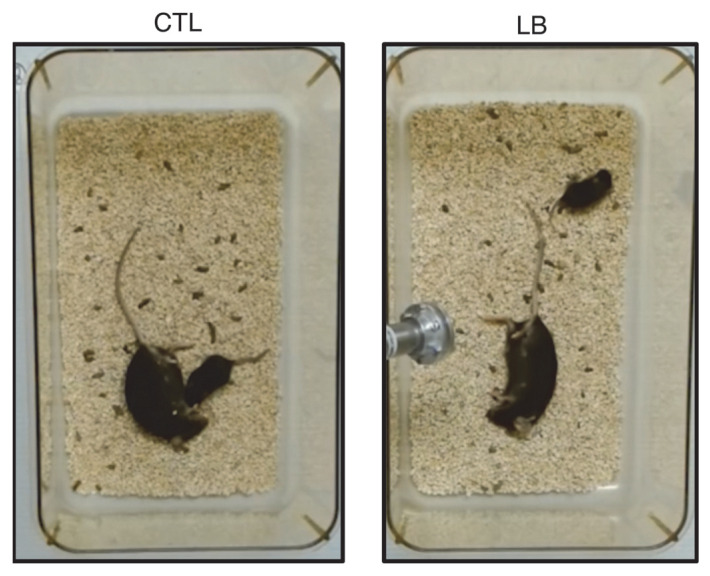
Thirteen-day-old control (CTL) and limited bedding (LB) pups were placed with their anesthetized dam for 5 min to assess proximity-seeking behavior and record ultrasonic vocalizations (USVs; analogous to distress calls) using a specialized microphone (shown in the LB image). Dams were anesthetized to observe pup-directed behavior and vocalizations. CTL pups exhibited the expected approach behavior toward the dam, whereas LB pups did not. Additional methodological details, quantification, and videos of this behavior are available in our previous work [59].
